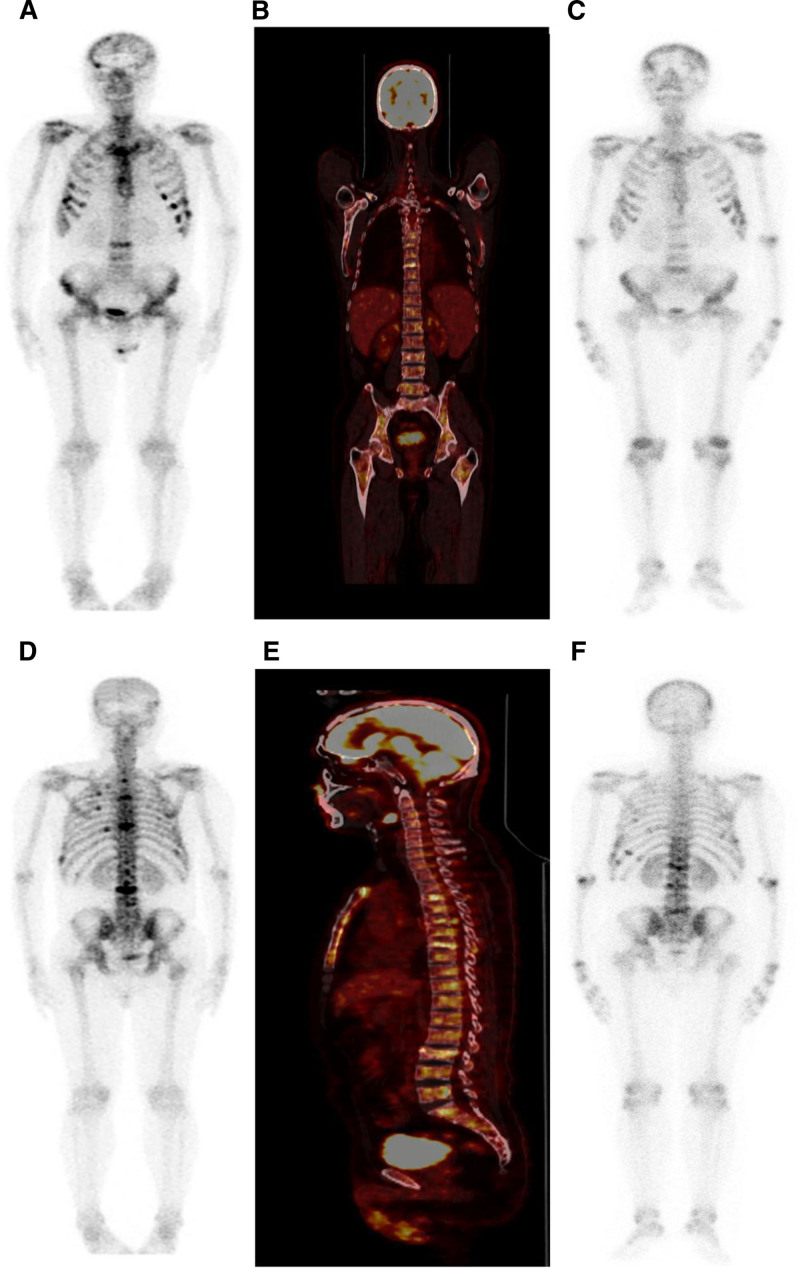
(A and D) Bone scan suggested the involvement of the skull, bilateral scapulae, sternum, and multiple branches of ribs on January 21, 2022. (B and E) PET/CT did not detect any lesions other than those in the bones. (C and F) Bone scans showed fewer lesions in the thoracic spine than before, but a few new lesions in the lumbar spine and ribs on July 27, 2022.
